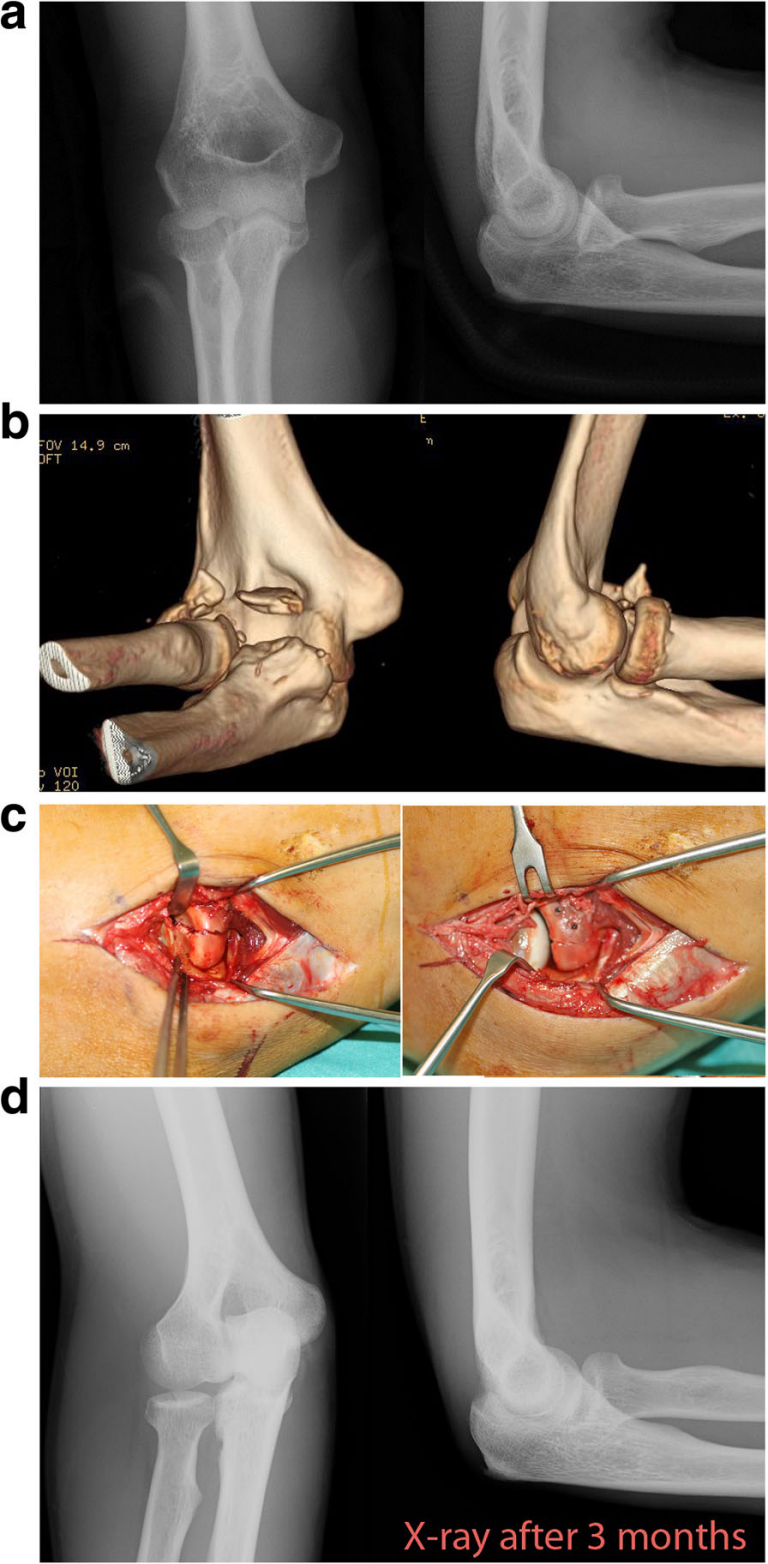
a X-rays showing a Mason type II fracture. b 3D-computed tomography evaluation confirming a partial articular fracture with high displacement of the fractured fragments. c Intra-operative view showing the reduction and fixation using 2 absorbable pins. d X-rays performed after 3 months showing healing of the fracture without signs of secondary displacement of the fragments
